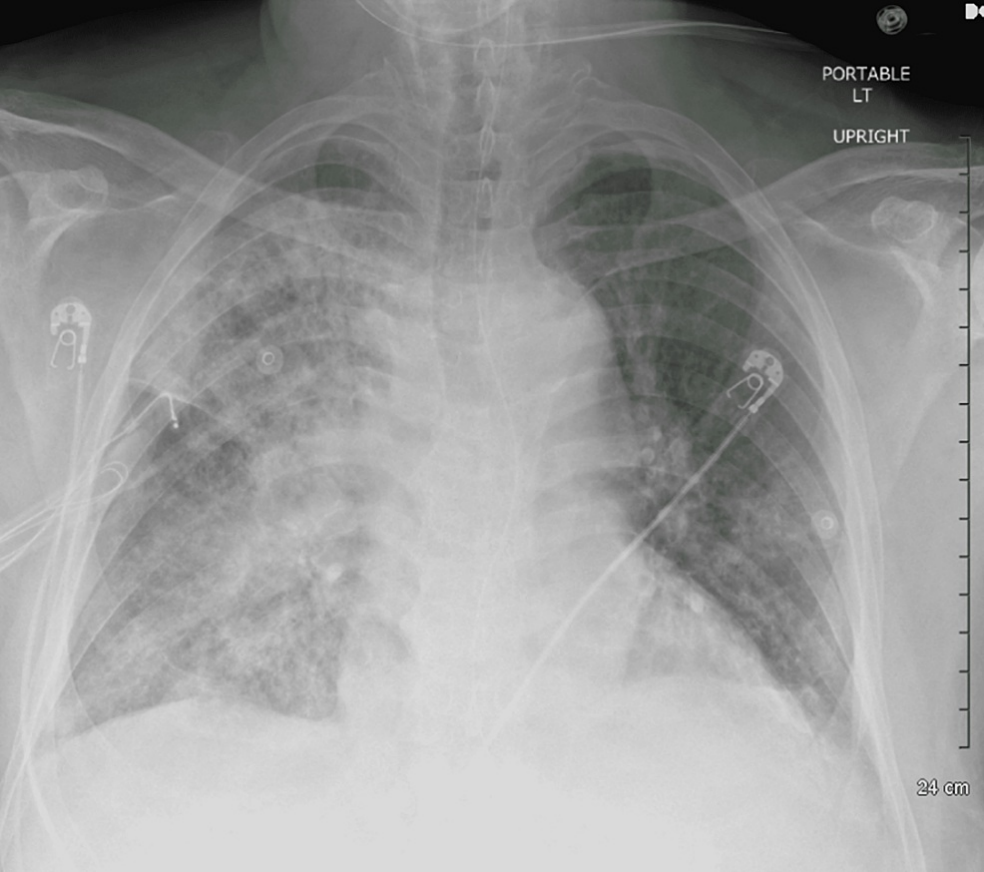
Chest X-ray showing diffuse right lung consolidation
